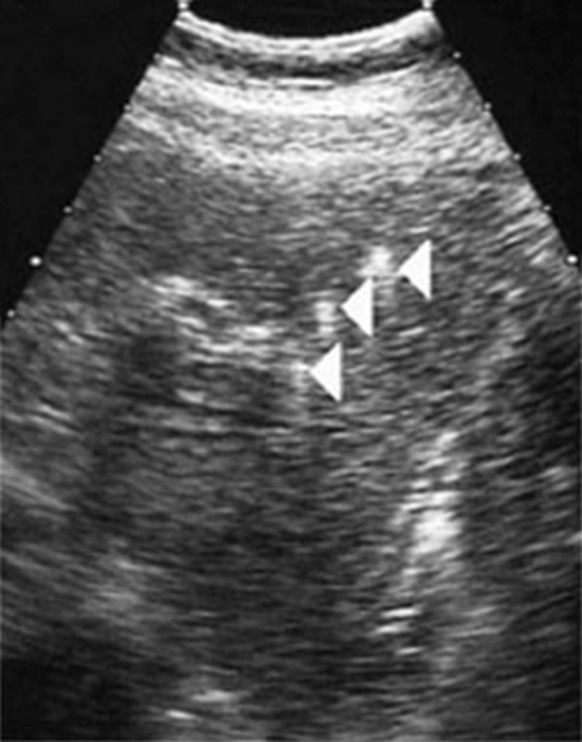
Ultrasound images of intrahepatic hyperechoic foci in patients with the LPAC syndrome. In these patients, a careful US examination detected intrahepatic hyperechoic foci with diffuse topography compatible with lipid deposits along the luminal surface of the intrahepatic biliary tree (Arrows). These multiple dots, less than 1 mm in diameter, cast short echogenic trail without acoustic shadows and looked like comet tails. They were typically distributed along the portal arborizations and may be associated with intrahepatic sludge or microlithiasis casting typical acoustic shadows.
